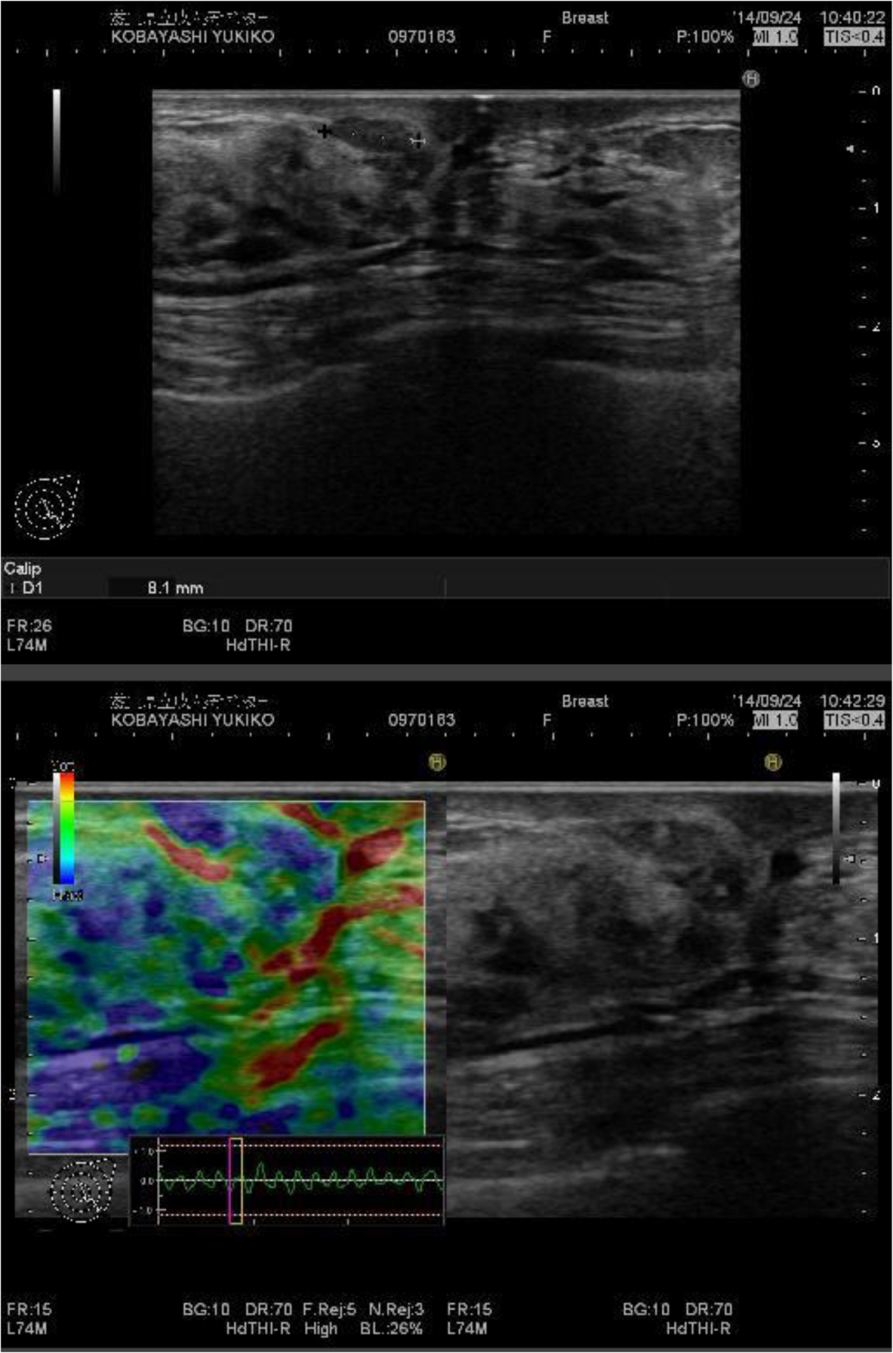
Ultrasonographic findings. Breast ultrasonogram showed an irregular low echoic area in the inner lower quadrant. No blood flows were present in the low echoic area, and elastography revealed that the area was soft unlike breast cancer
